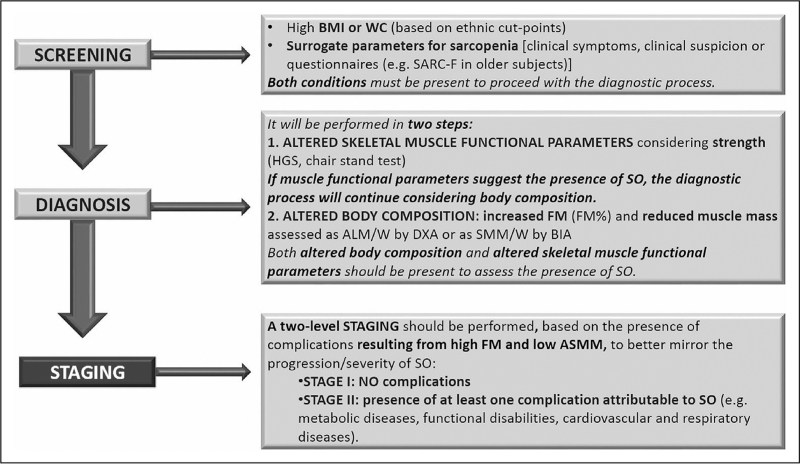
Diagnostic procedure for the assessment of sarcopenic obesity according to the ESPEN-EASO algorithm. ALM/W, appendicular lean mass adjusted to body weight; BIA, bioelectrical Impedance analysis; DXA, dual x-ray absorptiometry; FM, fat mass; HGS, handgrip strength; SARC-F, strength, assistance with walking, rising from a chair, climbing stairs and falls SMM/W, total skeletal muscle mass adjusted by weight; SO, sarcopenic obesity; WC, waist circumference. Previously published by Donini *et al.*[5^▪^].
